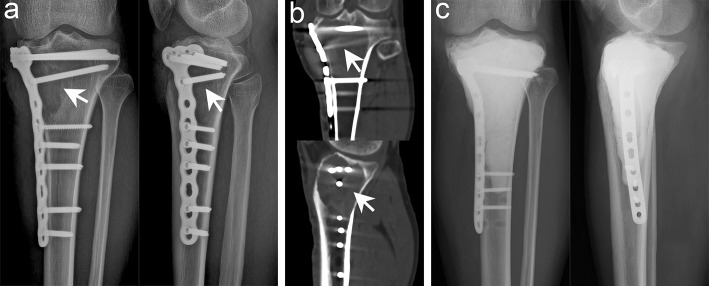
Typical imaging manifestations of patients with local recurrence treated with extended curettage. (a) Anteroposterior and lateral radiographs showed that the proximal and lateral parts of the right tibia had obvious osteolytic foci at the original site. (b) Computed tomography (CT) scan confirmed low-density osteolytic changes in the original site. (c) Extended curettage, cement filling, and subchondral bone grafting were performed after relapse of GCTB was confirmed by biopsy (white arrow points to the lesion).
